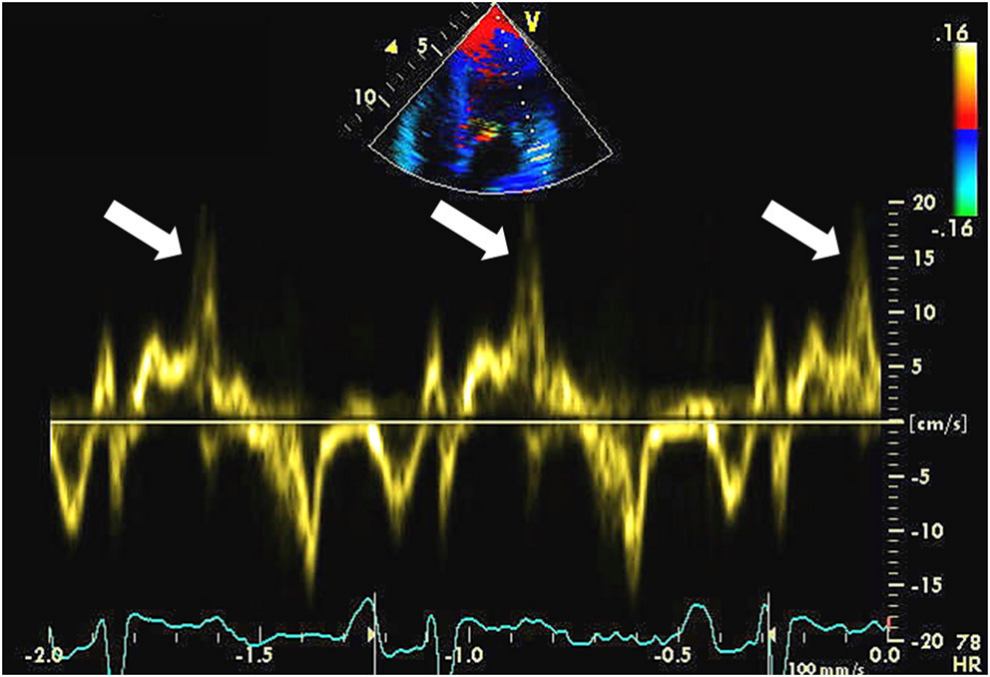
An echocardiographic example of the “Pickelhaube sign” which reflects increased velocity at the lateral mitral annulus in mid-systole to late-systole. The velocity spike is denoted by a white arrow.
